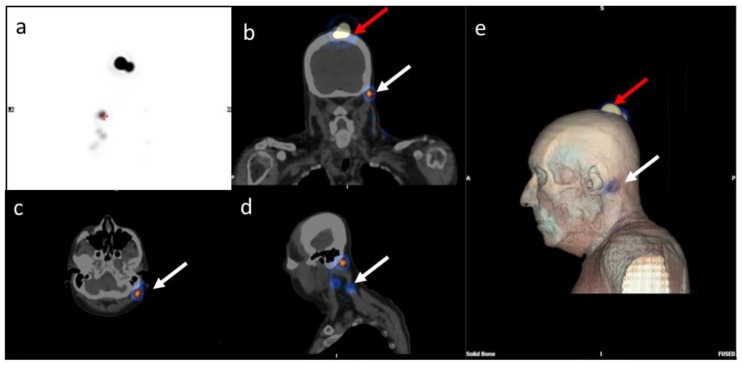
99mTc-serum albumin lymphoscintigraphy in a 65 year-old patient with scalp primary infiltrative head and neck cutaneous melanoma (red arrows). (a) maximum intensity projection (MIP), (b) coronal, (c) trans-axial, and (d) sagittal fused images, single-photon emission computed tomography/computed tomography (SPECT/CT). (e) A 3D reconstruction shows the sentinel lymph nodes drained to the preauricular and cervical level II-IV. Three sentinel lymph node (SLNs) were detected. (white arrows).
